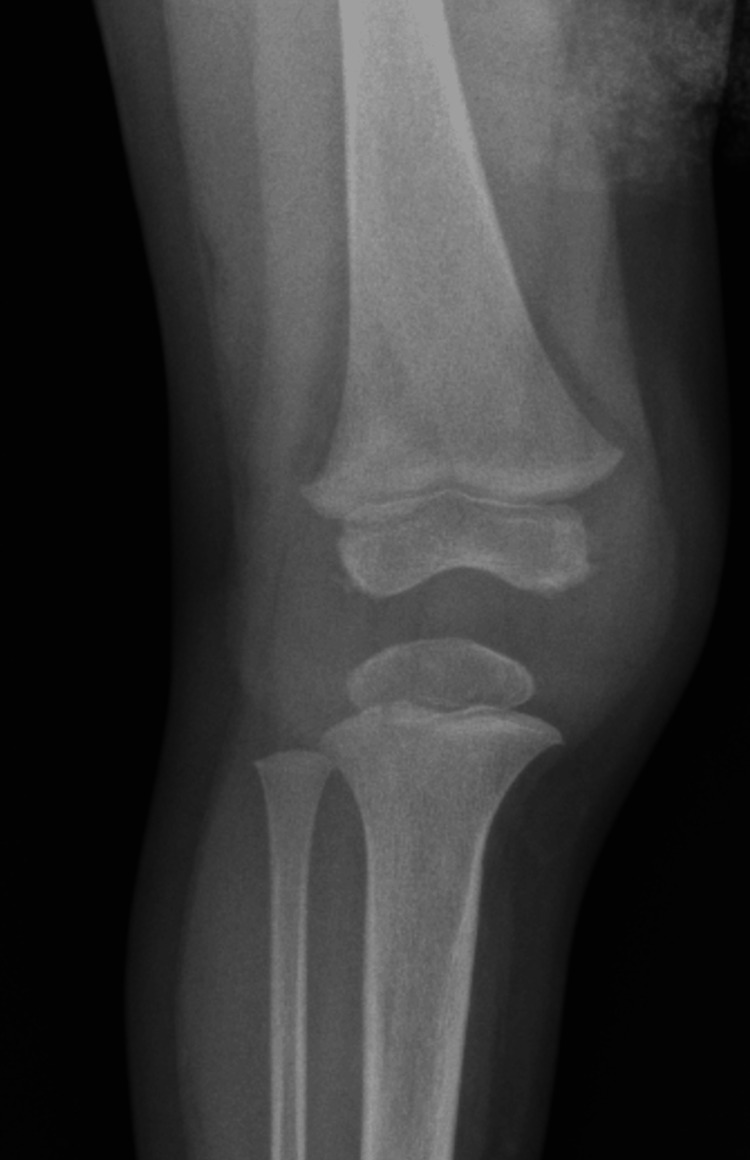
Anterior-Posterior Radiograph of the Right Knee Showing No Abnormalities
